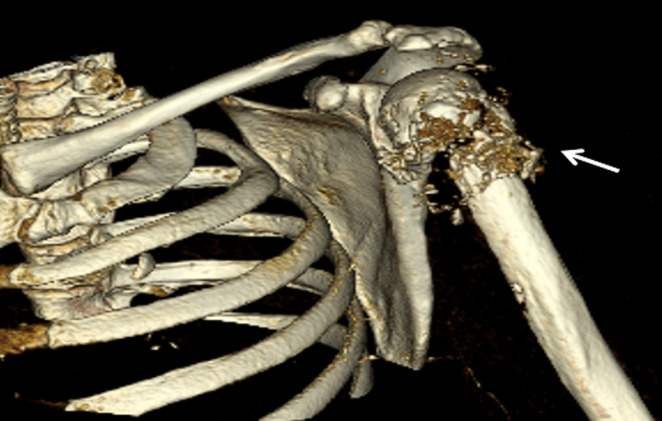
CT 3-D imaging of left shoulder showing pathological fracture of proximal left humerus with areas of lysis and cortical destruction
